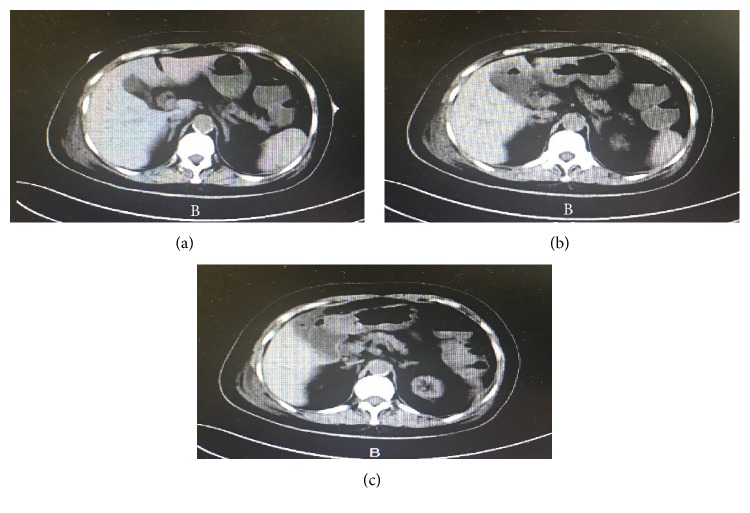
(a–c) Abdominal CT of another patient with the low fecal elastase level (less than 100 *μ*g/g) showed atrophy of the pancreas.
